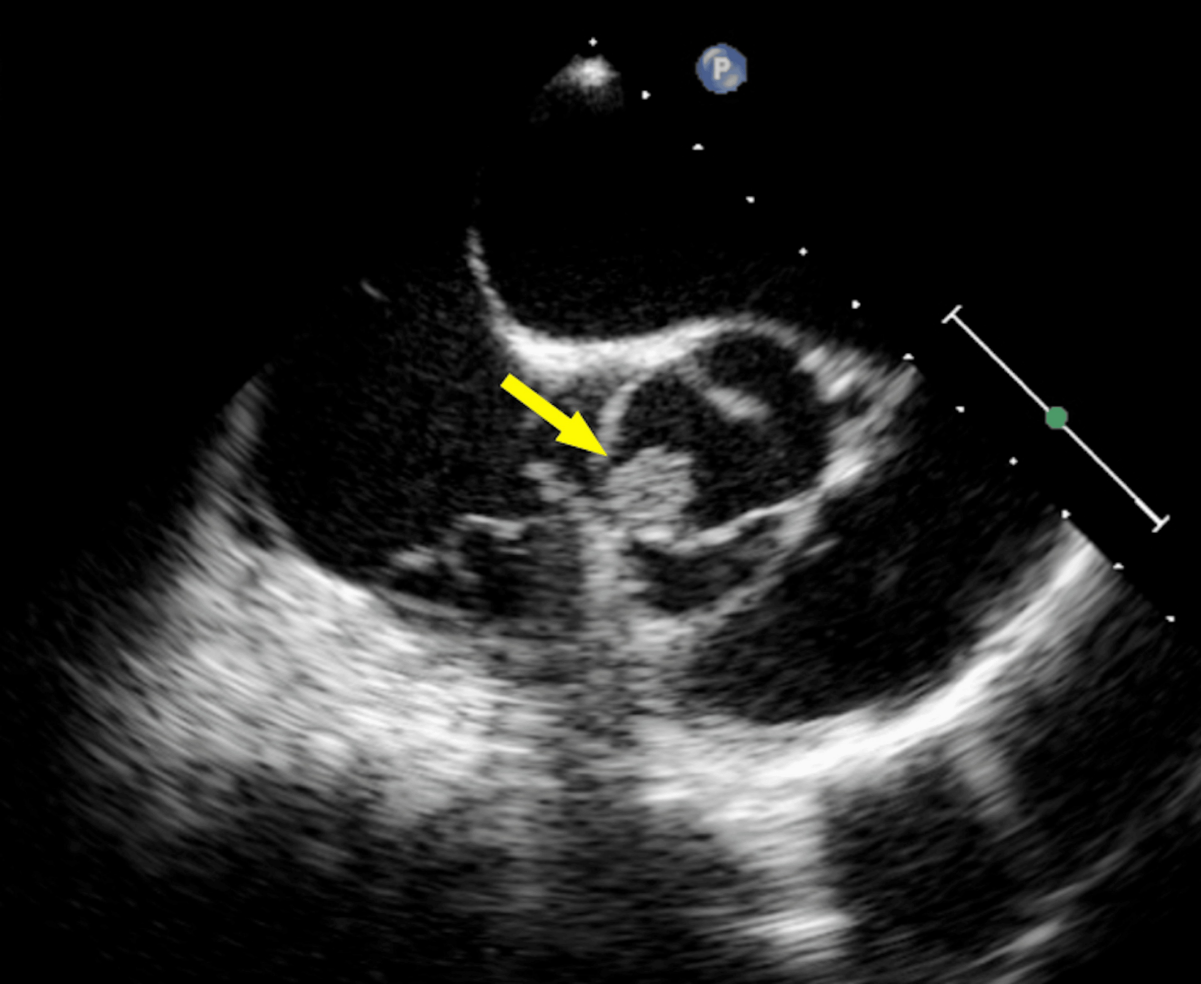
Transesophageal echocardiography at the initial hospital Vegetation is observed at the bicuspid aortic valve (yellow arrow).
